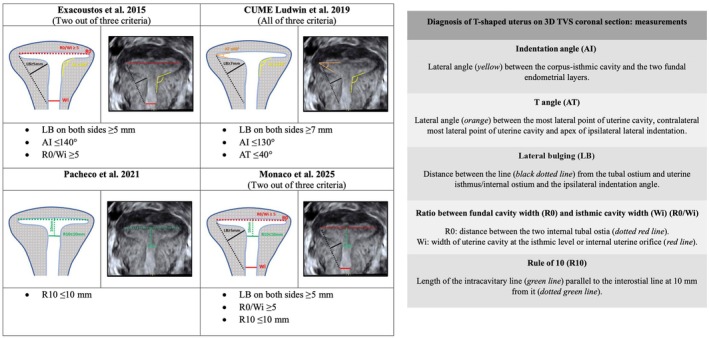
Diagnostic criteria proposed for the identification of T‐shaped uterus. Based on three‐dimensional (3D) transvaginal ultrasound image, these criteria differ in anatomical parameters and diagnostic thresholds.
